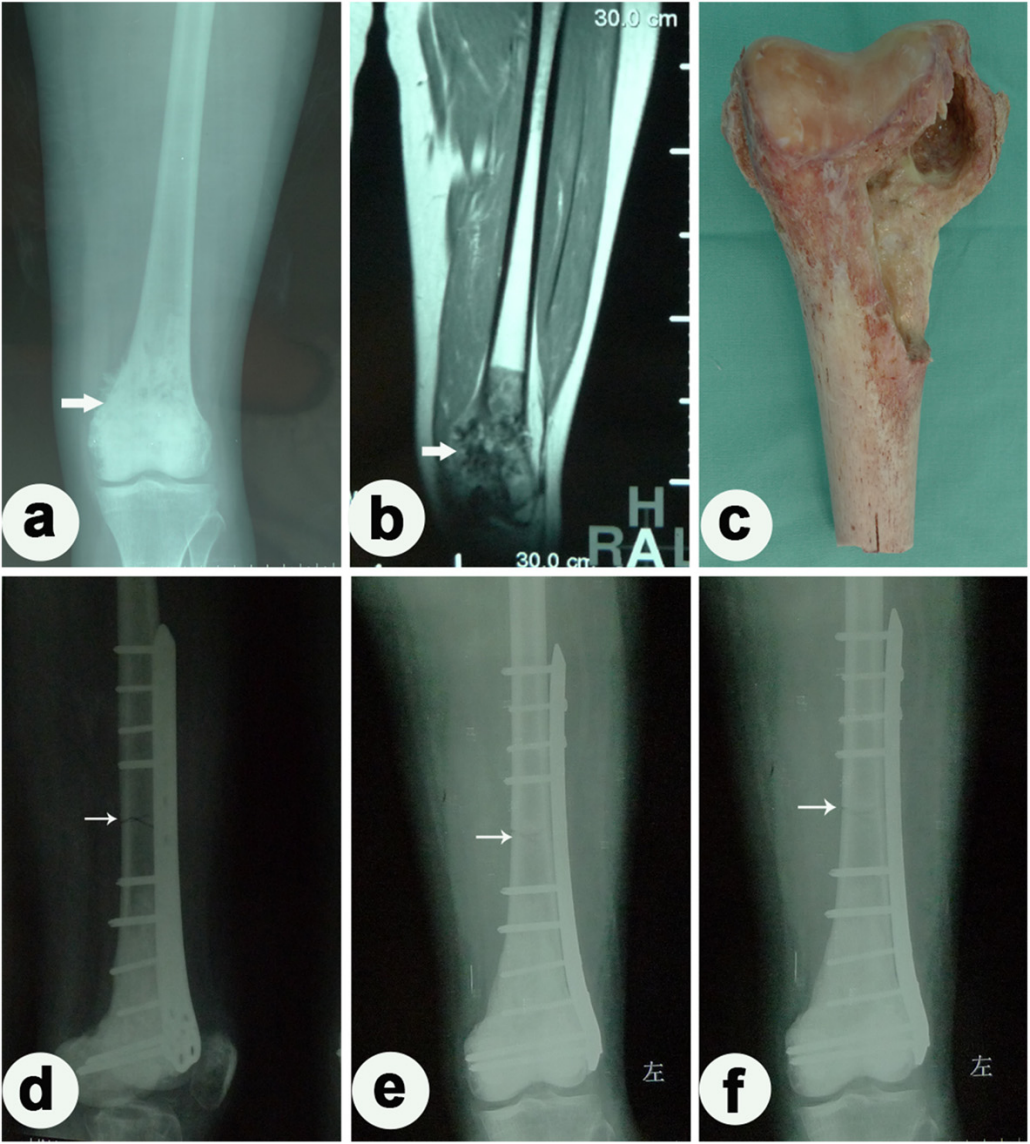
Case presentation II. Case 37: A 45-year-old woman was diagnosed as having chondrosarcoma in the distal of her left femur and was treated with wide resection and inactivated autograft using alcohol. a Plain radiography before surgery. b MRI before surgery. c Inactivated autograft by alcohol in surgery. d Three months after surgery. e One year after surgery. f Two years after surgery
